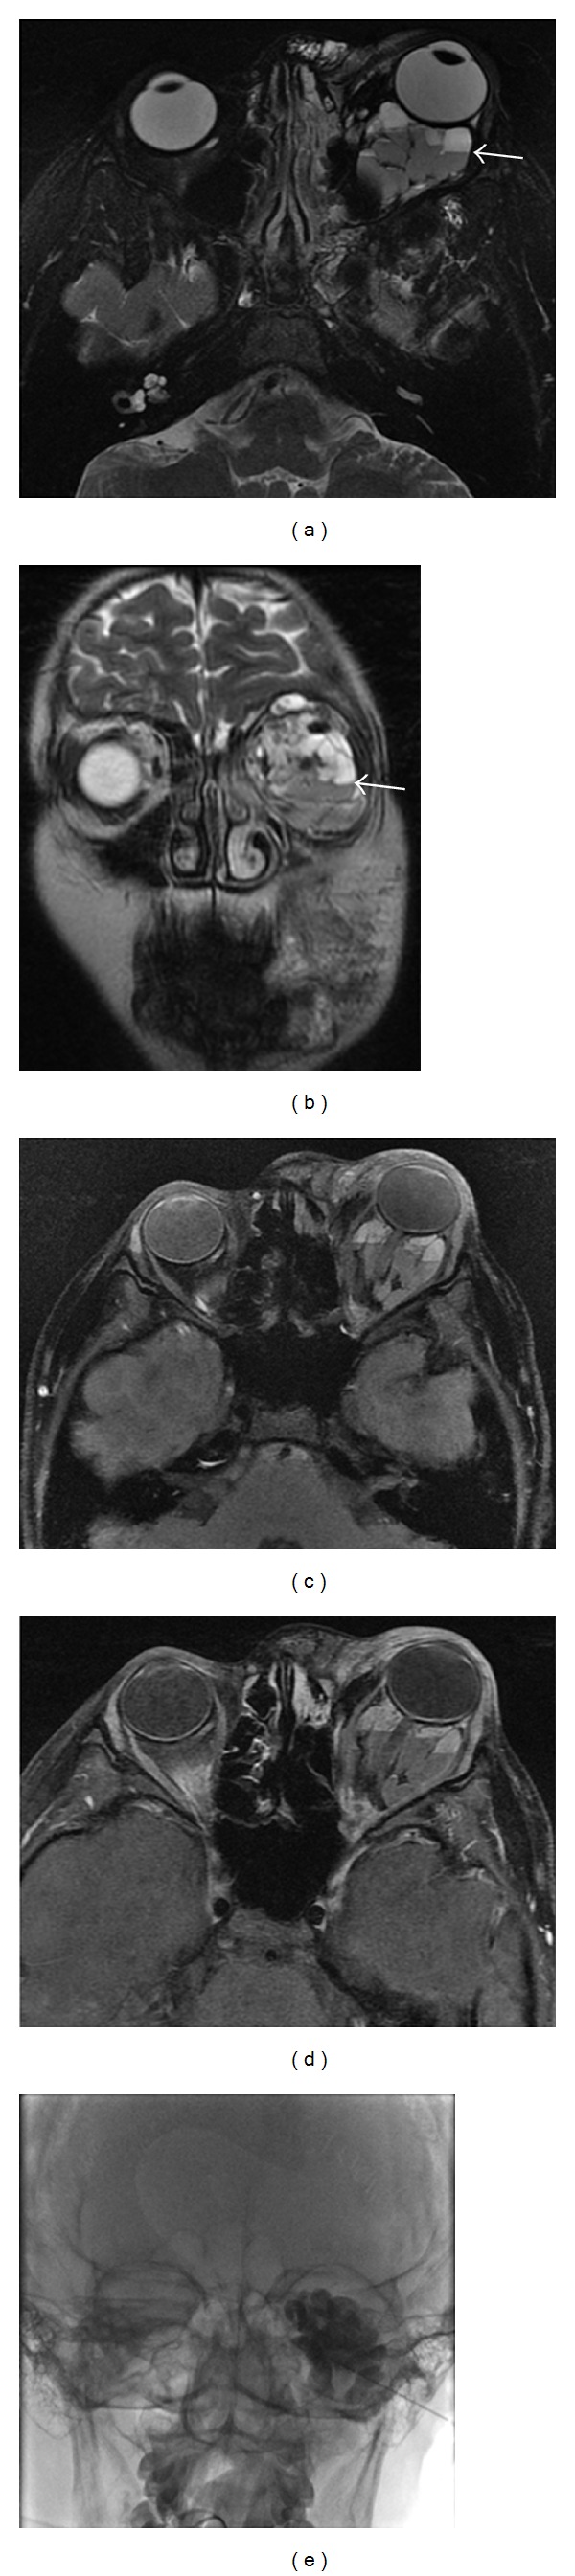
17 years old with acute worsening of left eye proptosis secondary to intralesional hemorrhage. MR axial T2-weighted fat-saturated (a) and coronal T2WI (b), axial T1-weighted fat-saturated image (c), and postcontrast T1-weighted fat-saturated image (d) showing a lobulated, trans-spatial mass with fluid-fluid levels, and trace rim enhancement (white arrows). The fluid-fluid levels are due to intralesional hemorrhage and are almost pathognomonic for a VLM. The lesion was treated with sclerotherapy using bleomycin and sodium tetradecyl sulfate (e).
